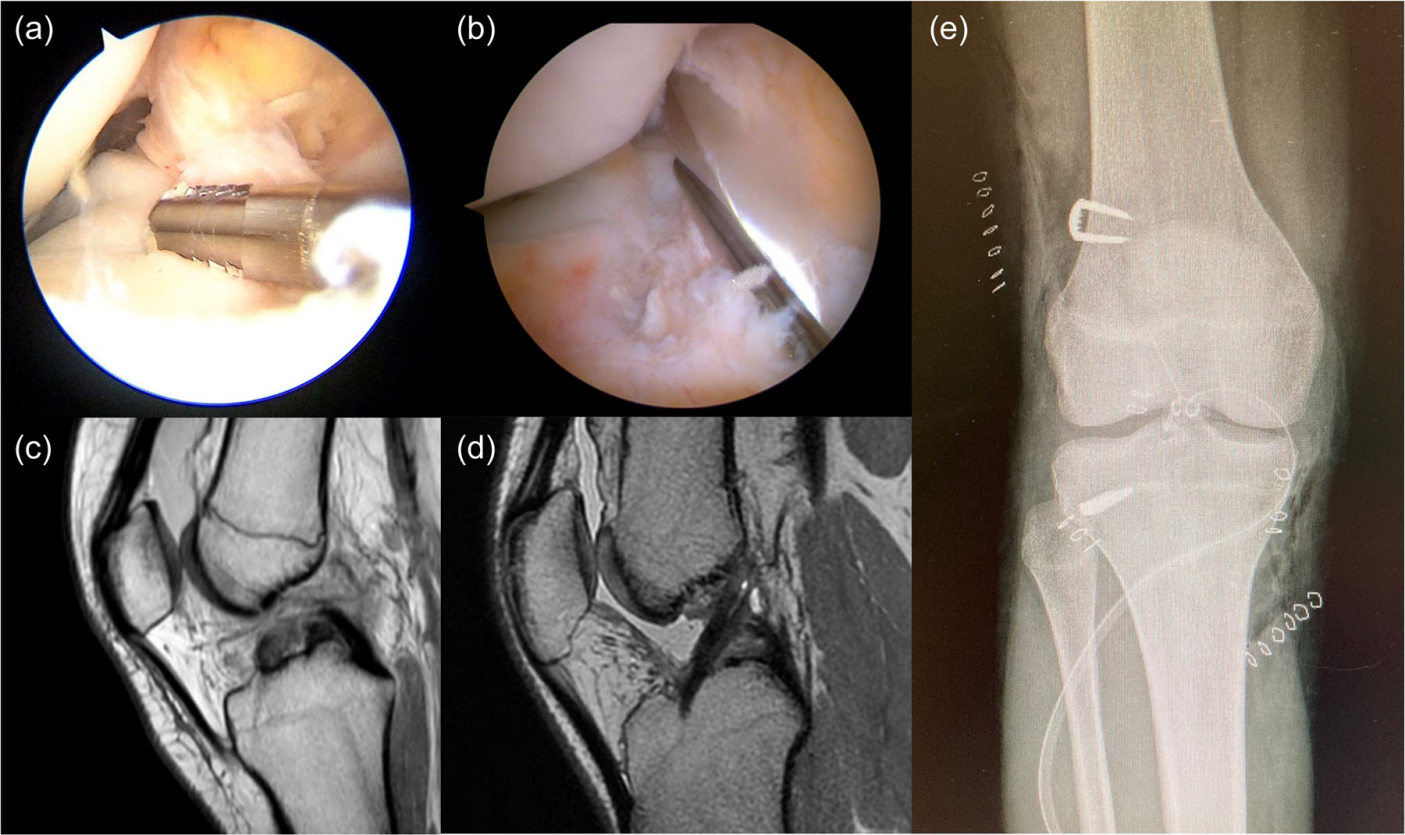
Male basketball player with chronological age of 15.8 years and skeletal age of 16 years treated with trans‐physeal over‐the‐top ACL reconstruction. Arthroscopic vision with the empty notch due to ACL injury (a) and after reconstruction with hamstring graft (b). Sagittal pre‐operative MRI shows the absence of the ACL (c) and 12‐month MRI shows the graft with a good ipointense signal (d). Antero‐posterior radiograph shows no conflict of the staples with the growth plates (e). ACL, anterior cruciate ligament.
